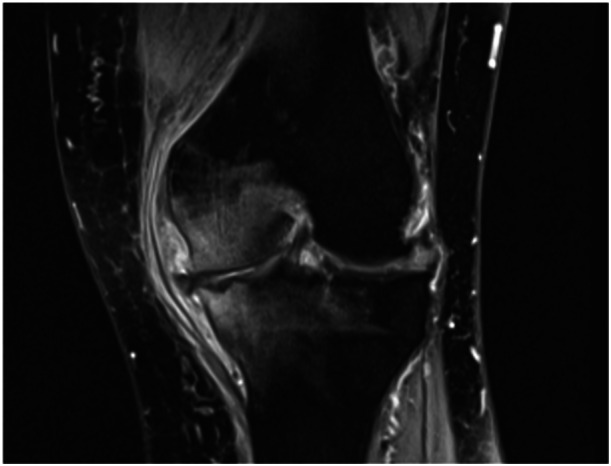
Coronal view in T2‐weighted fat‐suppressed magnetic resonance images demonstrating bone marrow oedema in the medial tibia and femur. Marked reduction of articular space, cartilage thickening, maceration and subluxation of the medial meniscus are present, indicating degenerative change of the knee. Lateral meniscopathy and chondropathy, less marked than the medial compartment, are shown. Soft tissue thickening with local synovial reaction that imprints and dislocates the capsuloligamentous compartment (1.5 T scanner; Magnetom Espree, Siemens).
